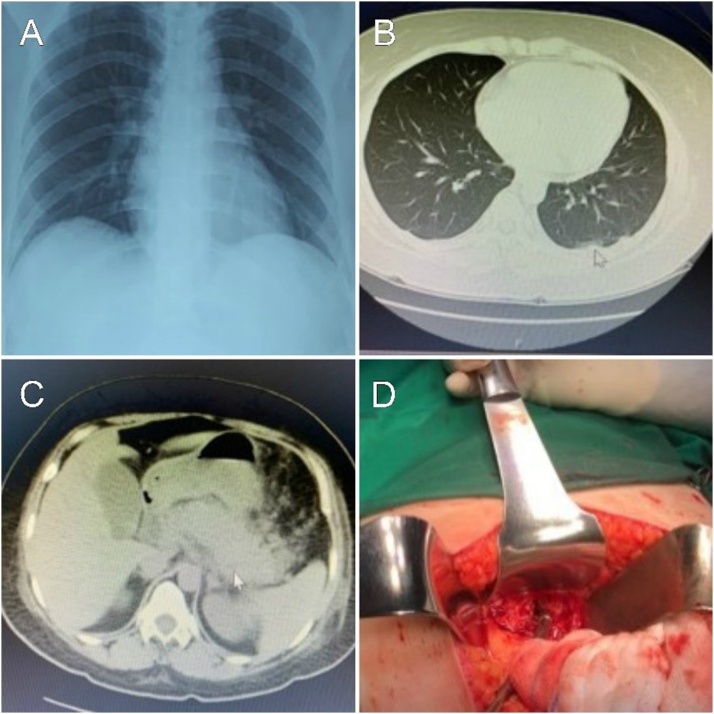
A) Chest X-ray showed normal lung and heart, B) Chest MSCT showed pleuritis, C) Abdominal MSCT showed pancreatitis necroticans, and D) Intraoperative finding of pancreatitis necroticans.
